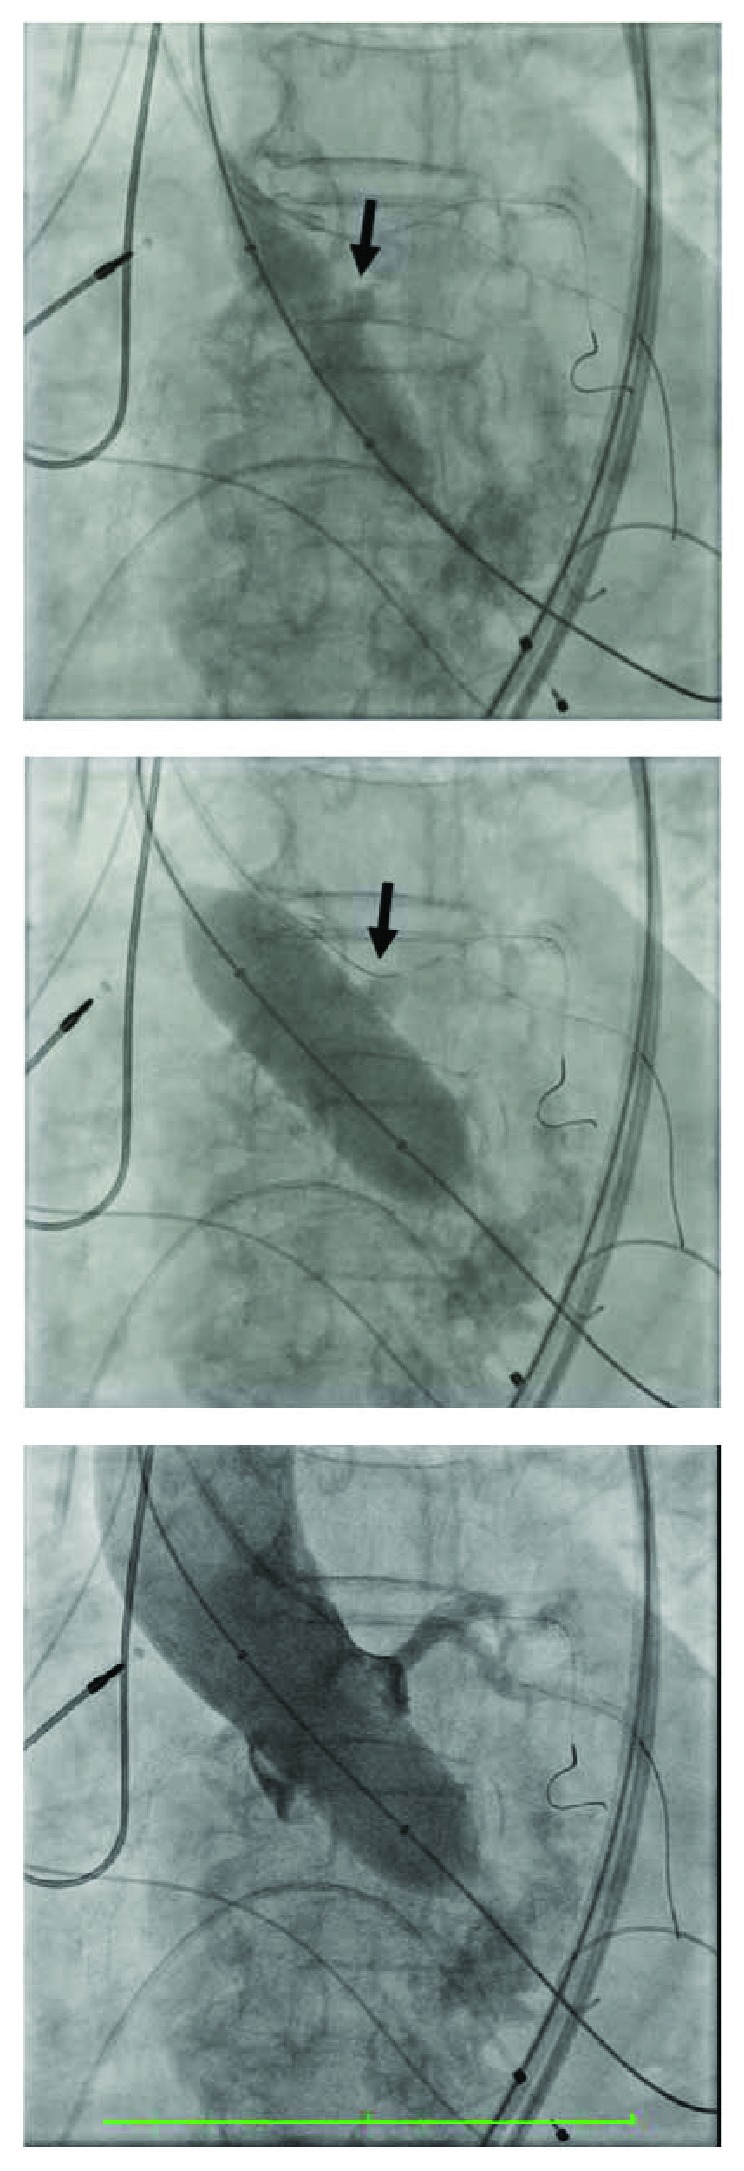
Balloon aortic valvuloplasty, notice the deformed wires and the large piece of calcium in the left cusp moved right over the left main coronary artery (arrows). Simultaneous aortic root injection showing decreased coronary flow TIMI-2. Echocardiogram demonstrated severe aortic stenosis with mean aortic valve pressure gradient of 68.6 mmHg and peak velocity of 5.15 m/s.
